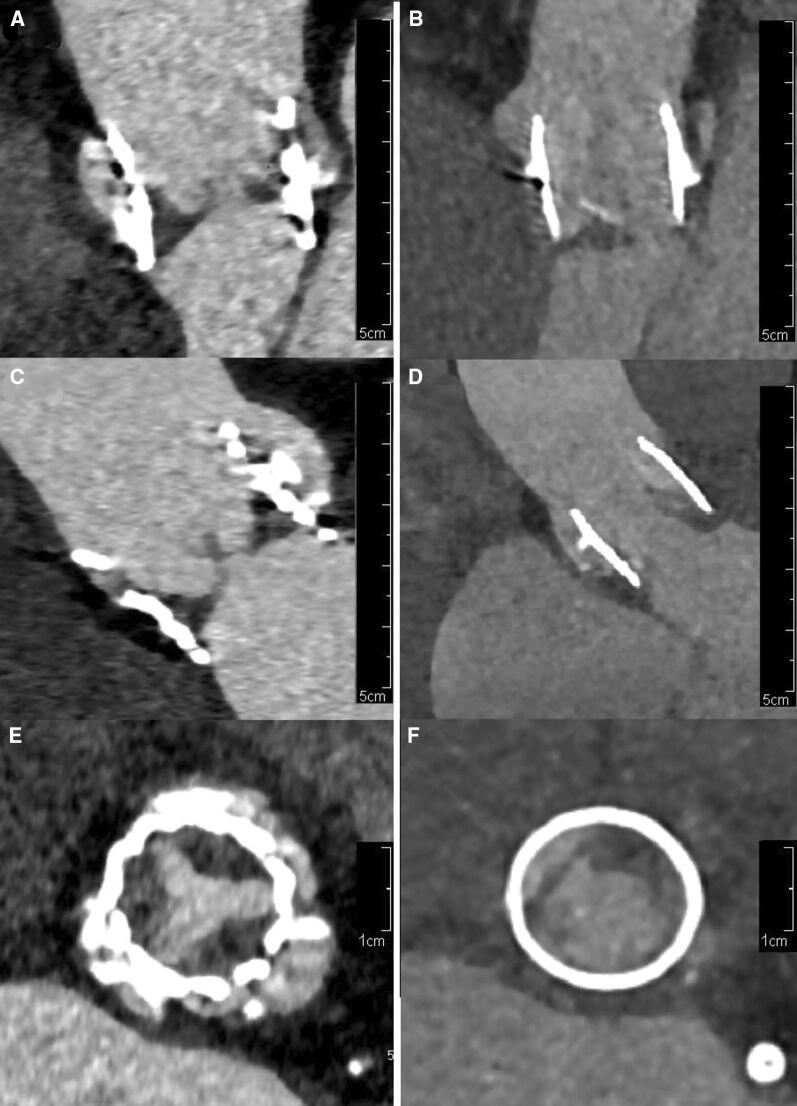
CTA images of a Sapiens valve with signs of leaflet thrombosis (A, C, E) and a Lotus valve with signs of vegetation (B, D, F)
